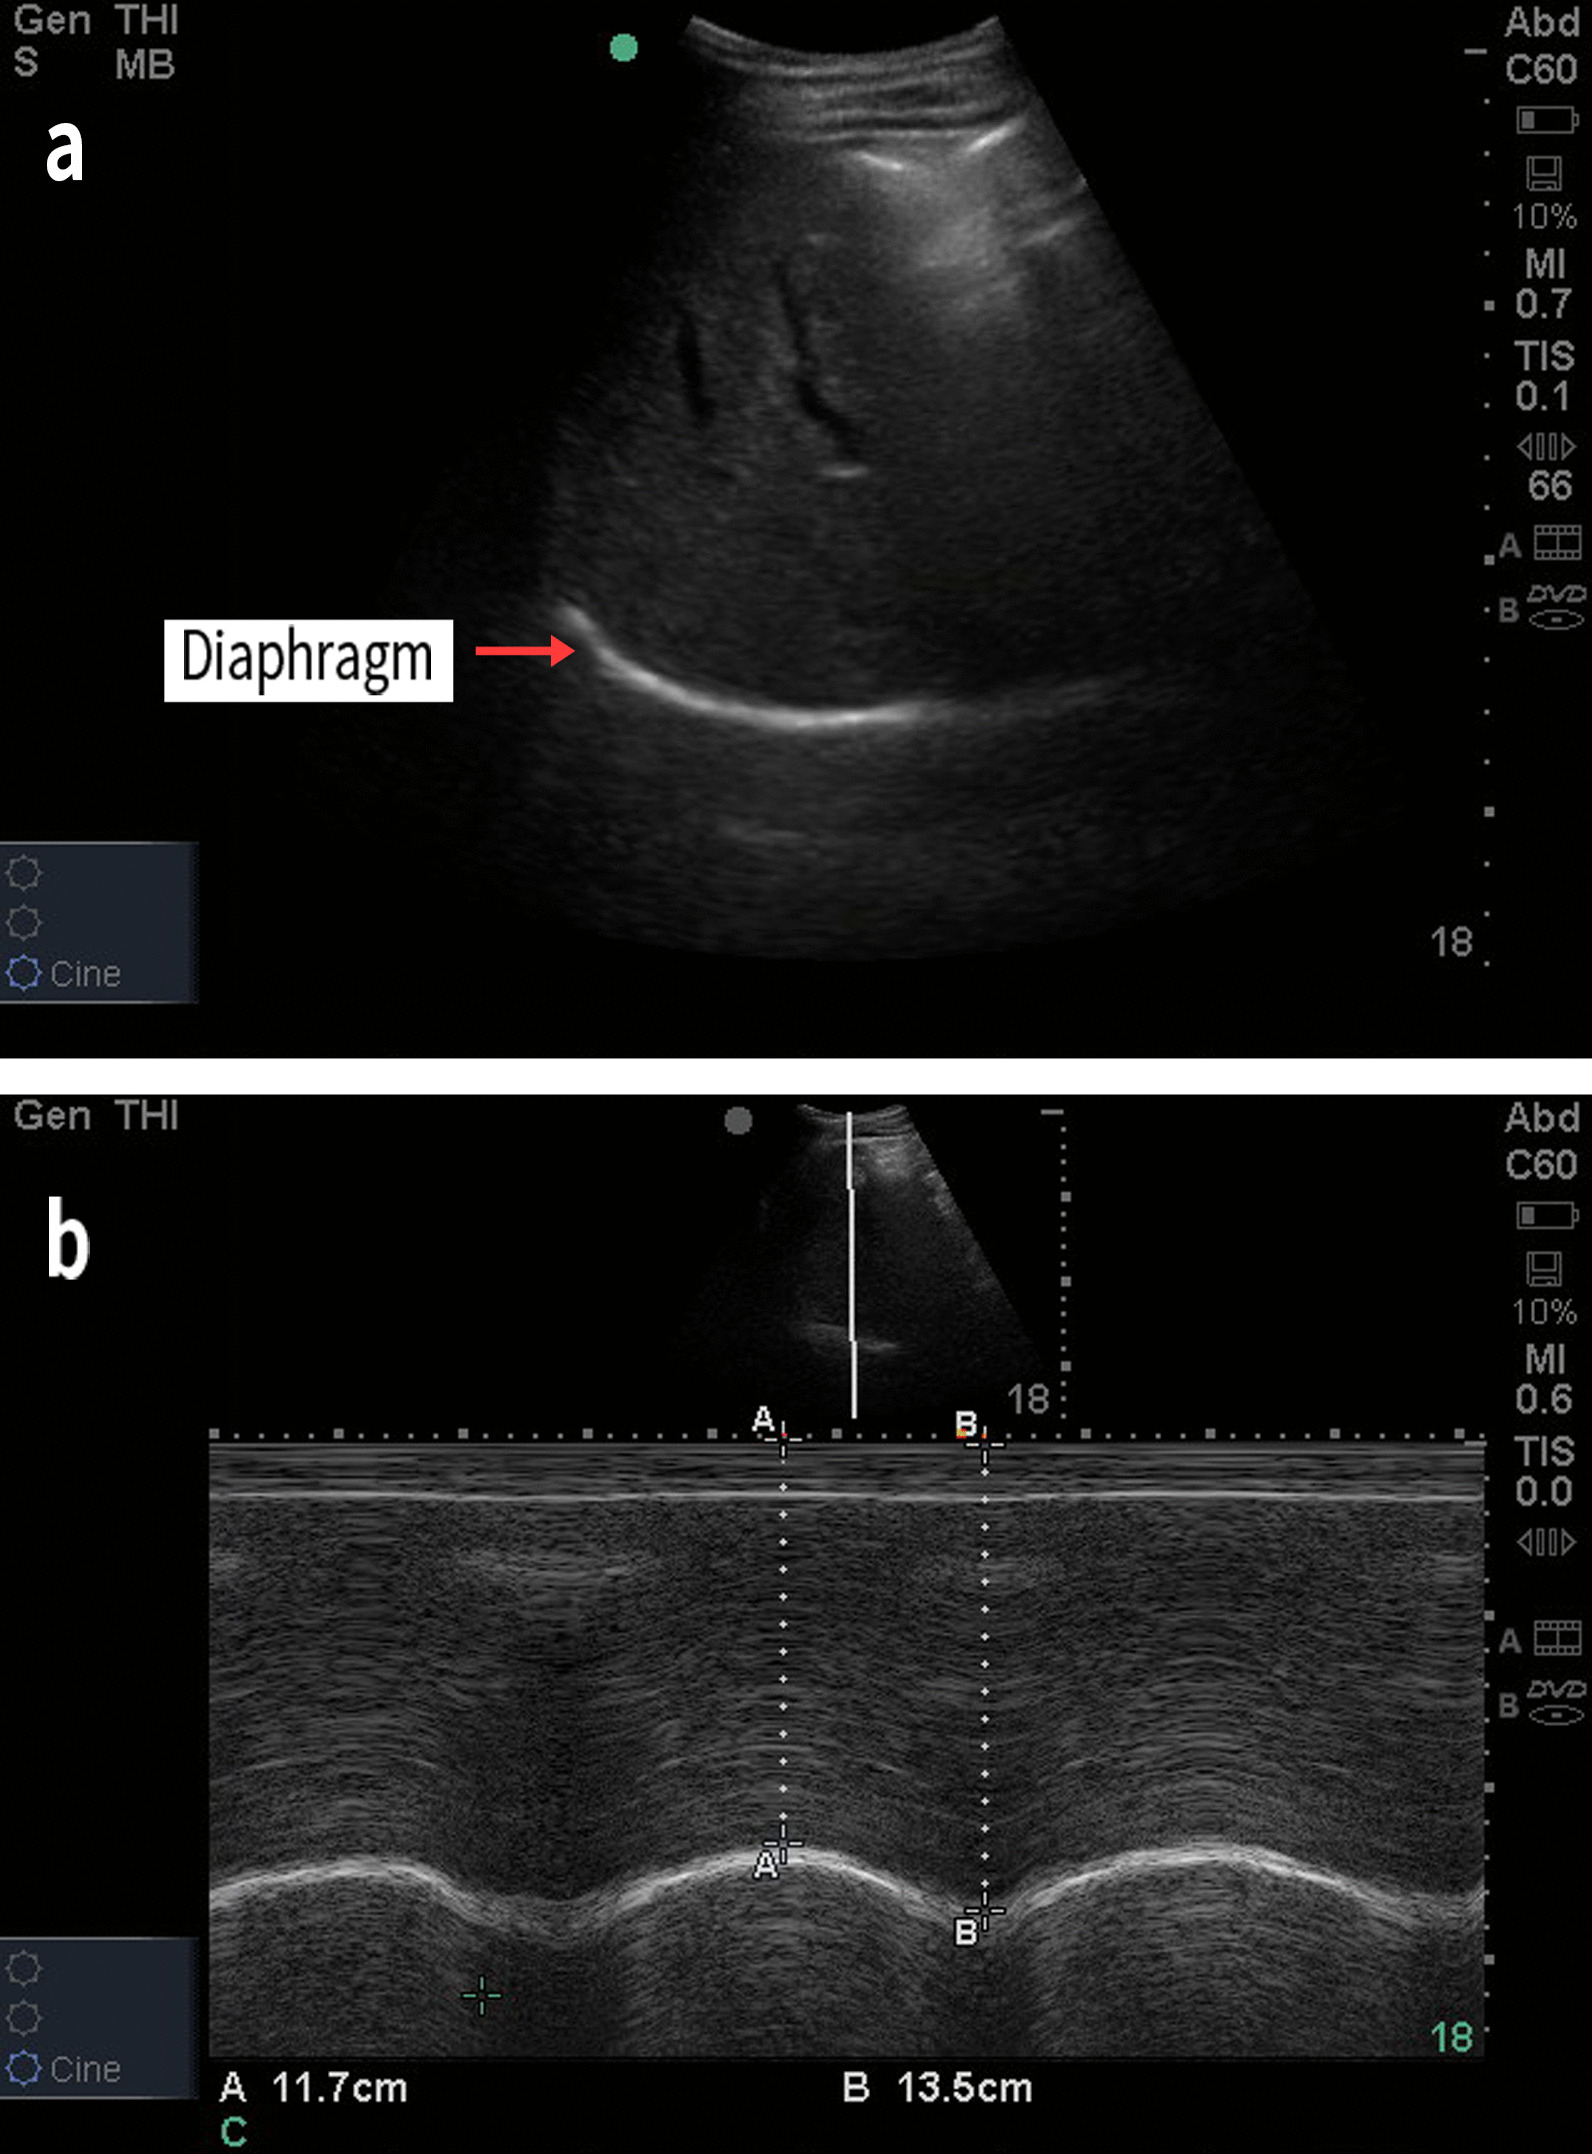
Diaphragmatic excursion (DE) measurement. a B-mode diaphragmatic ultrasonography. The bright line reflects the diaphragm. b M-mode diaphragmatic ultrasonography. DE during inspiration (A) and expiration (B) can be calculated according to B–A. In this image, DE was calculated as: 13.5 − 11.7 = 1.8 cm
